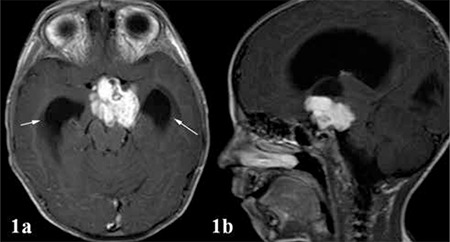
n the T1-weighted axial (a) and sagittal (b) sections a contrast-enhancing suprasellar mass with solid and cystic components is shown. Hydrocephalus is present due to compression by the mass (arrows)
